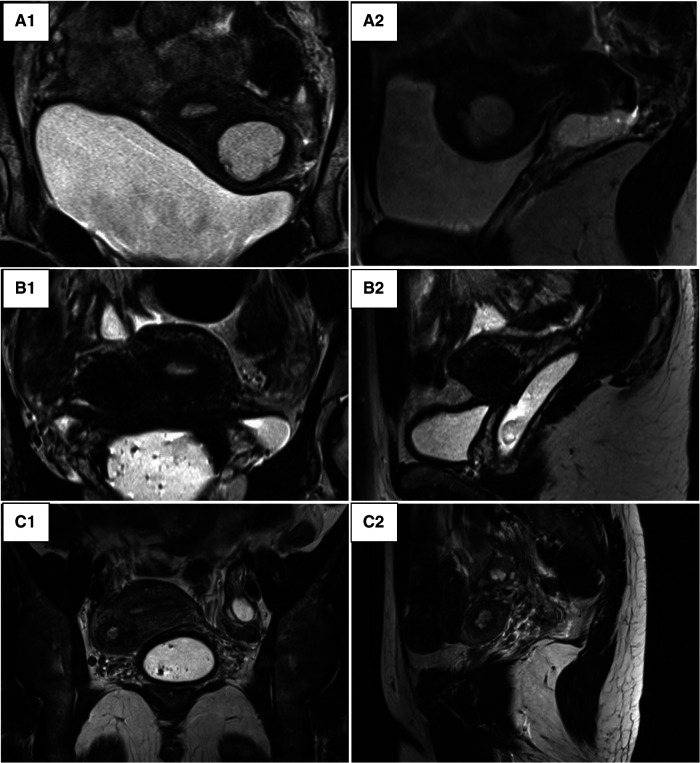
Pelvic MRI. (A) Patient 3_round mass in the left anterior myometrial wall suggestive of an accessory endometrial cavity within. (A1) T2-weighted coronal image. (A2) T2-weighted left lateral sagittal cut. (B) Patient 4_a round mass in the right anterior myometrial wall. (B1) T2-weighted coronal image. (B2) T2-weighted right lateral sagittal cut. (C) Patient 5_round mass in the right anterior myometrial wall. (C1) T2-weighted coronal image. (C2) T2-weighted right lateral sagittal cut.
